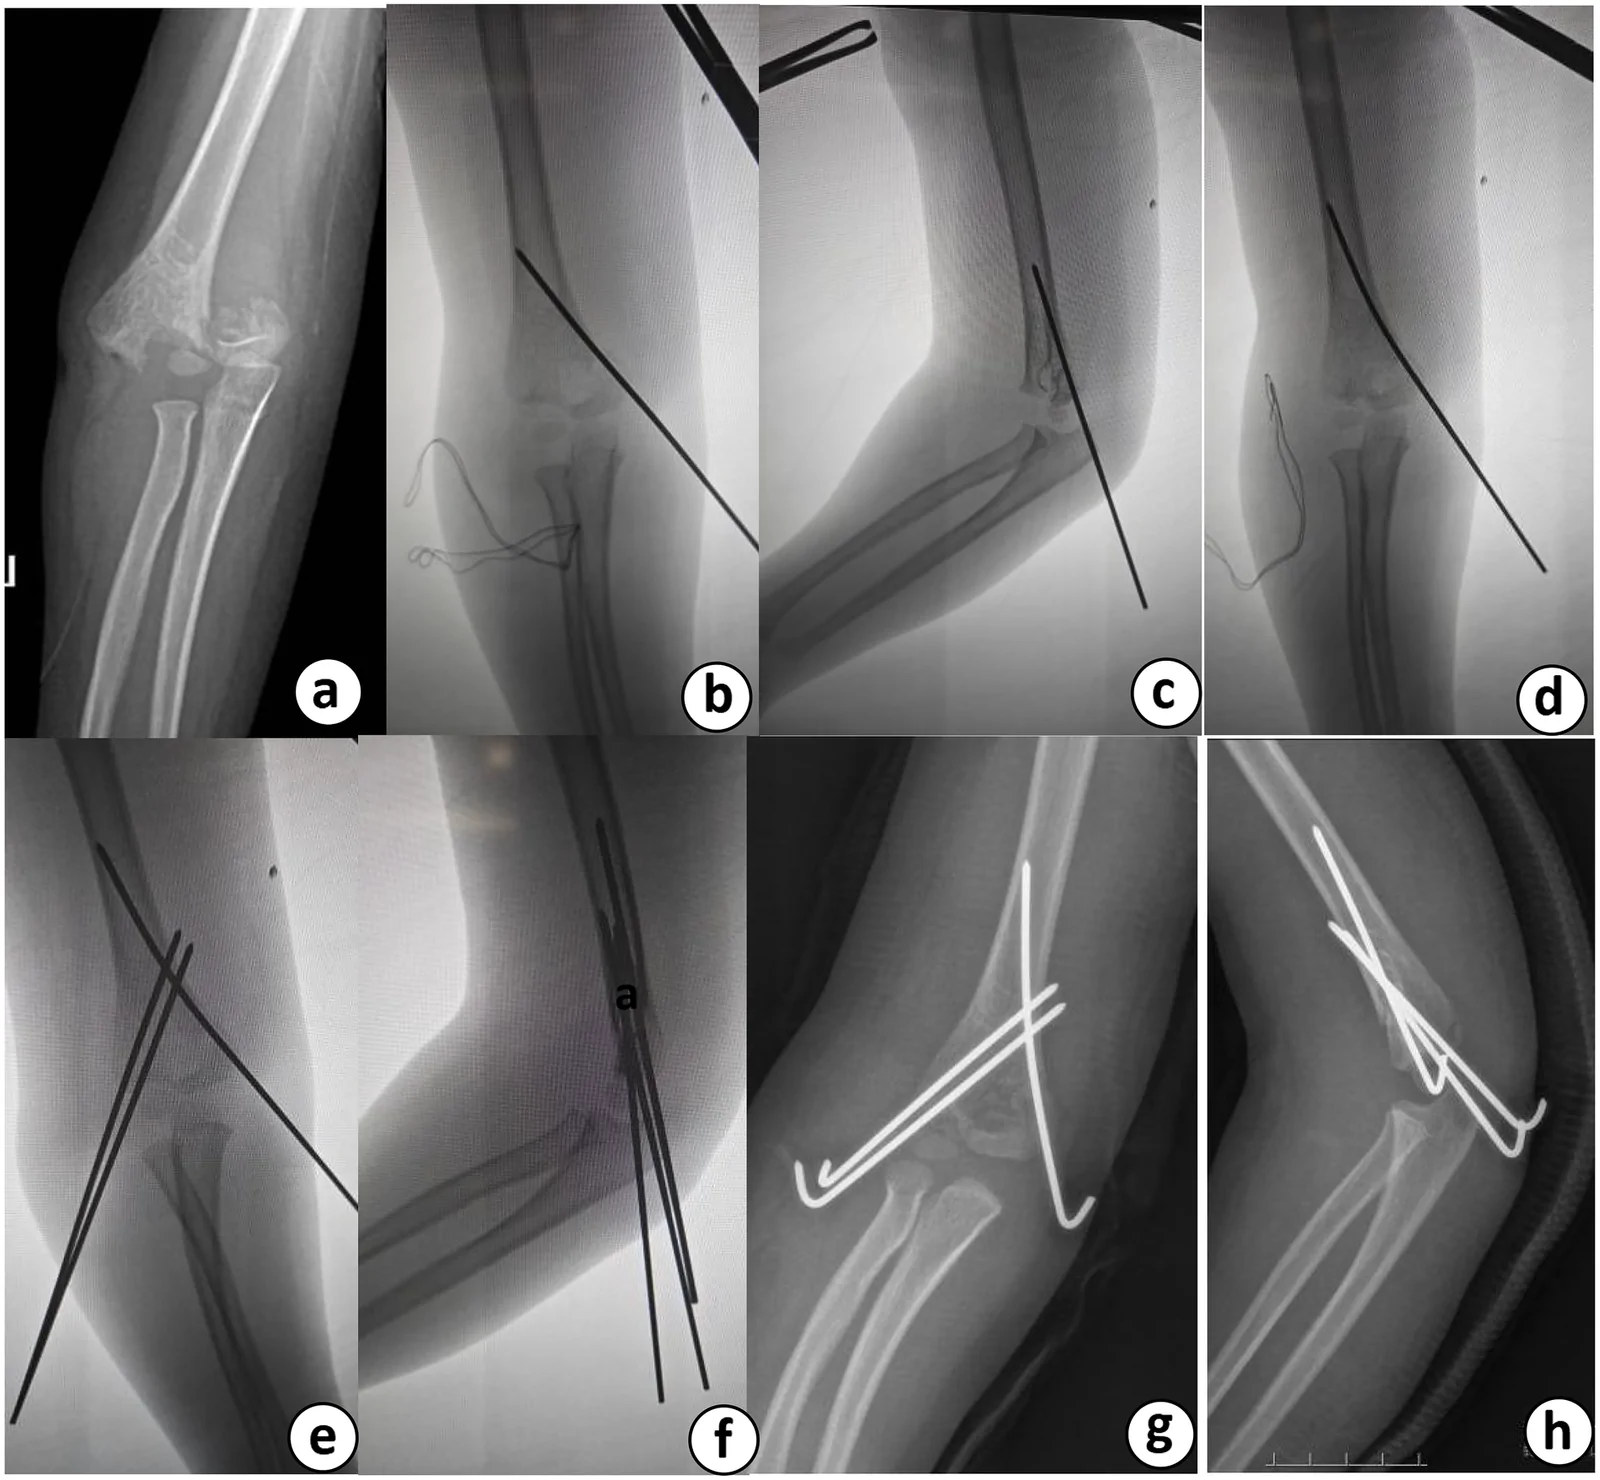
A 5-year-old boy with a Gartland type III supracondylar humeral fracture with an oblique fracture line running from superomedial to inferolateral. (a) Preoperative radiograph showing the fracture. (b,c) After initial closed manipulation, fluoroscopic localization of the ulnar entry point and K-wire insertion. (d) Gradual advancement of the ulnar K-wire into the medullary canal, correcting ulnar deviation. (e,f) Satisfactory reduction achieved, followed by radial-side K-wire fixation. (g,h) Postoperative AP and lateral radiographs of the elbow. This figure highlights the management of oblique fracture patterns, where the K-wire is inserted on the higher side of the fracture line to optimize compressive stress and shear force direction.
